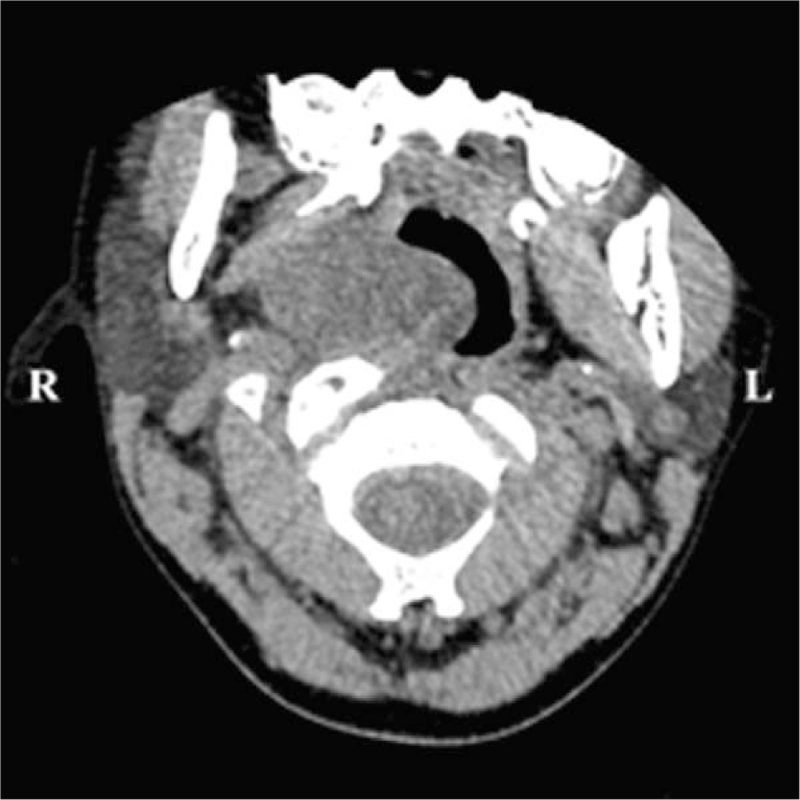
Preoperative plain CT scanning of the neck presents an oval soft tissue density shadow in the right pharyngeal wall, with CT value of about 31 HU.
